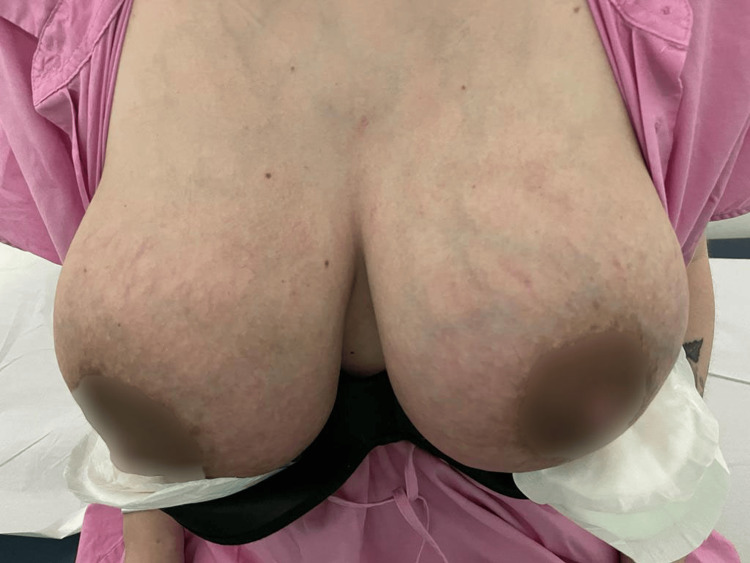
Day four of continuous therapeutic ultrasound
